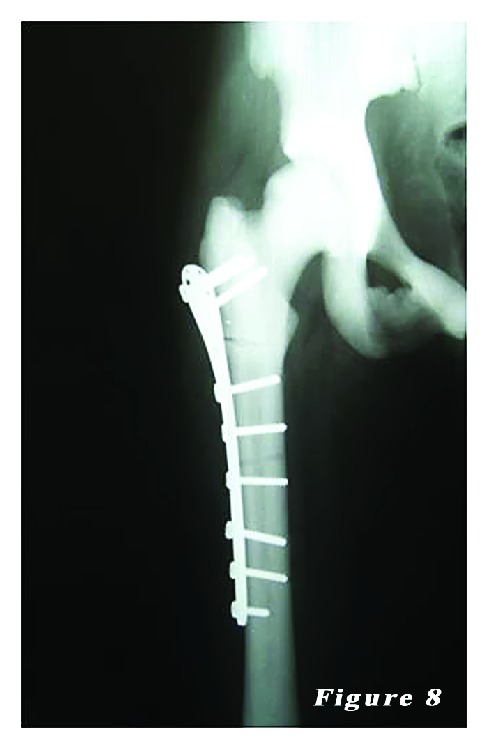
Postoperative right femur radiograph of the second patient.
